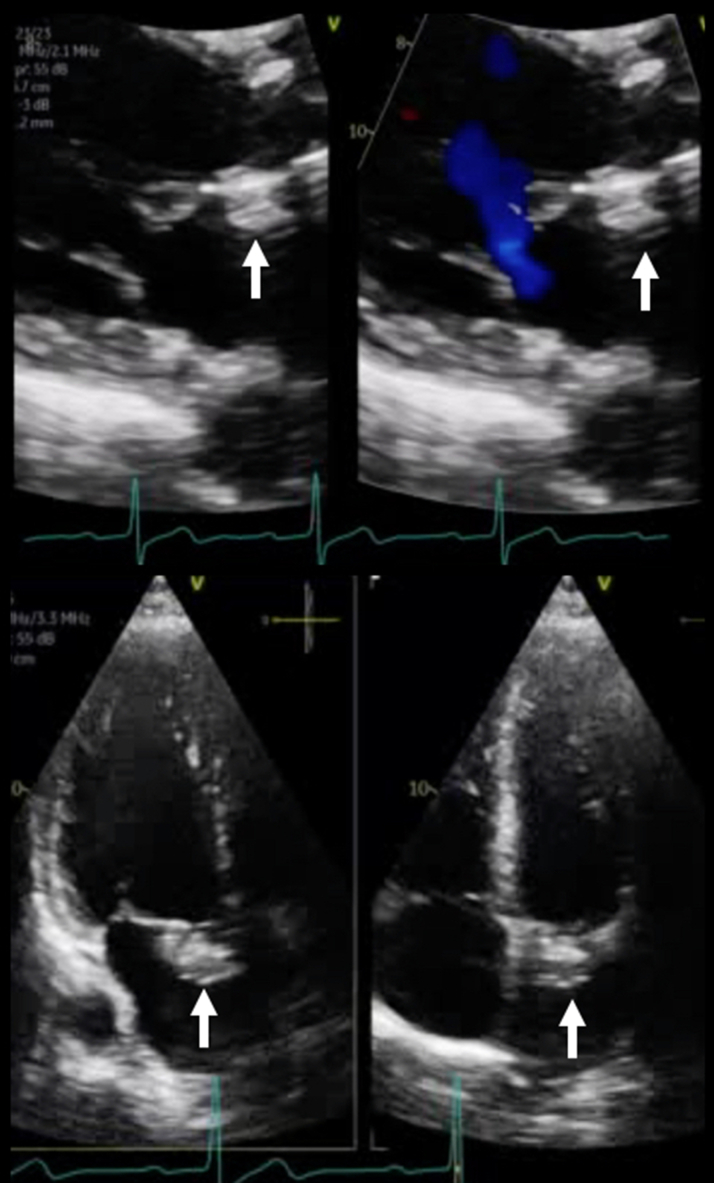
Follow-Up Transthoracic Echocardiography Five-month follow-up images confirm stable device position (arrows), with no residual leak and mild functional mitral regurgitation.
